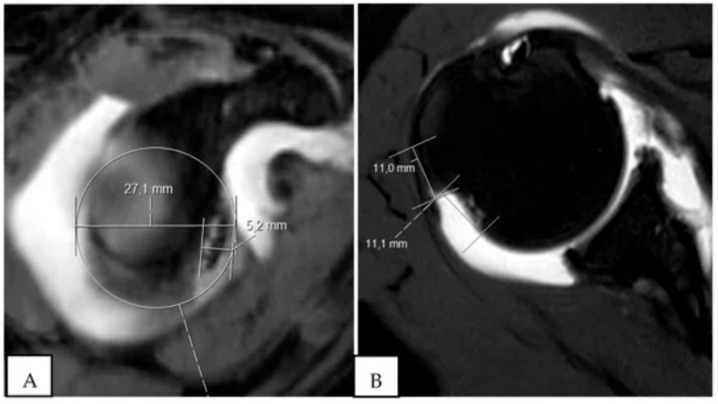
Off-track lesion on magnetic resonance imaging (MRI) arthrography. (A) In this case, the glenoid track is equal to 18.17 mm (27.1 – 5.2 × 0.83 = 18.17). (B) The Hill–Sachs index (HSI) is 22.1 mm (11.1 + 11). It is an off-track lesion because the glenoid track (18.17 mm) is less than the HSI (22.1 mm) [16].
